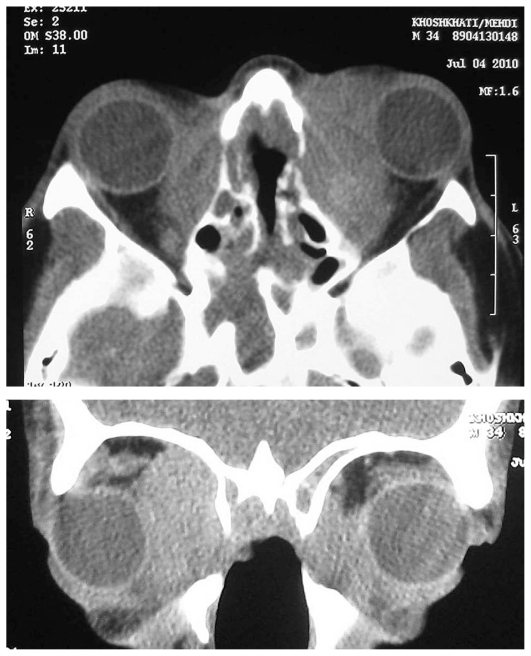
Axial (top) and coronal (bottom) sections on orbital computed tomography. Homogenous extraconal orbital mass lesions are seen on both sides adjacent to the medial walls. Bone erosion and destruction of the medial wall and nasal septum are also noted. The globes are displaced anteriorly and laterally especially on the left side.
